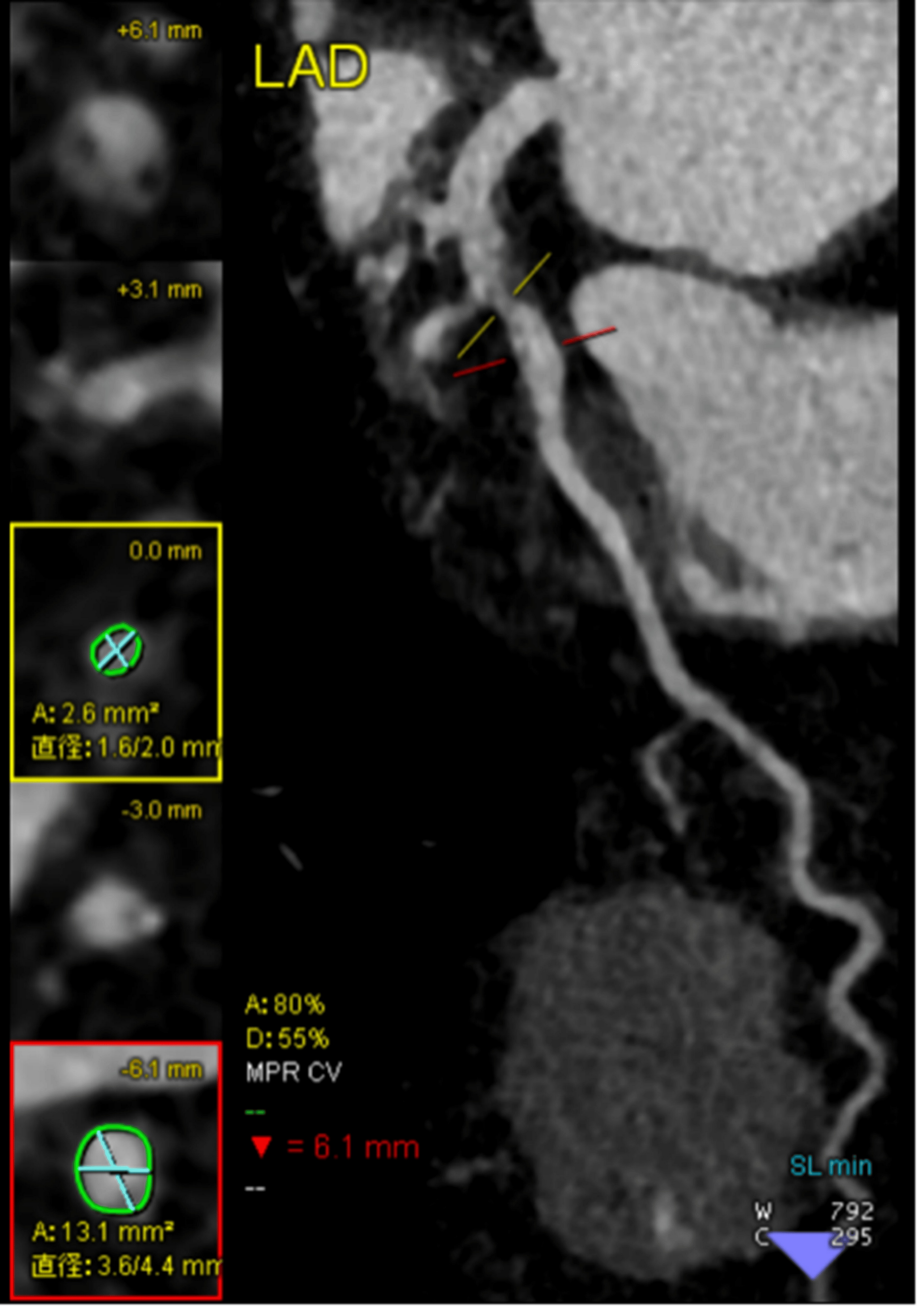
Left anterior descending artery (LAD) showing positive remodeling, punctate calcifications, and stenosis (80%) on coronary computed tomography angiography.
